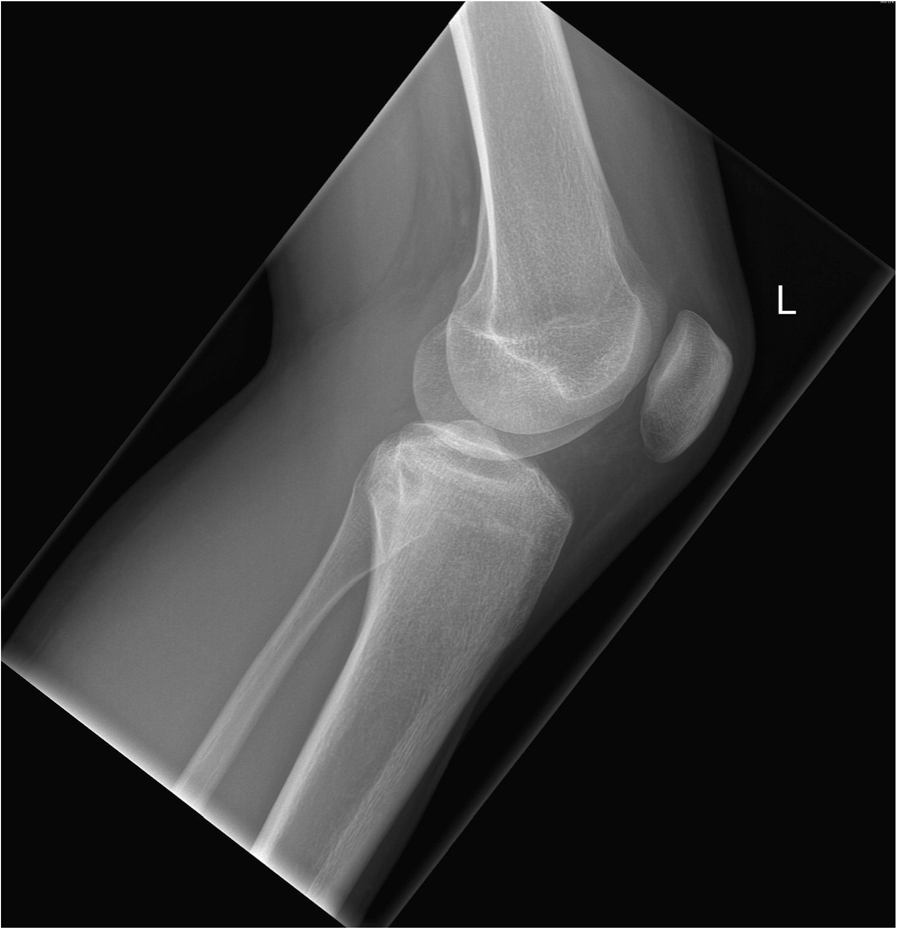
X-ray of the left knee (lateral view), taken at the first out-patient presentation
